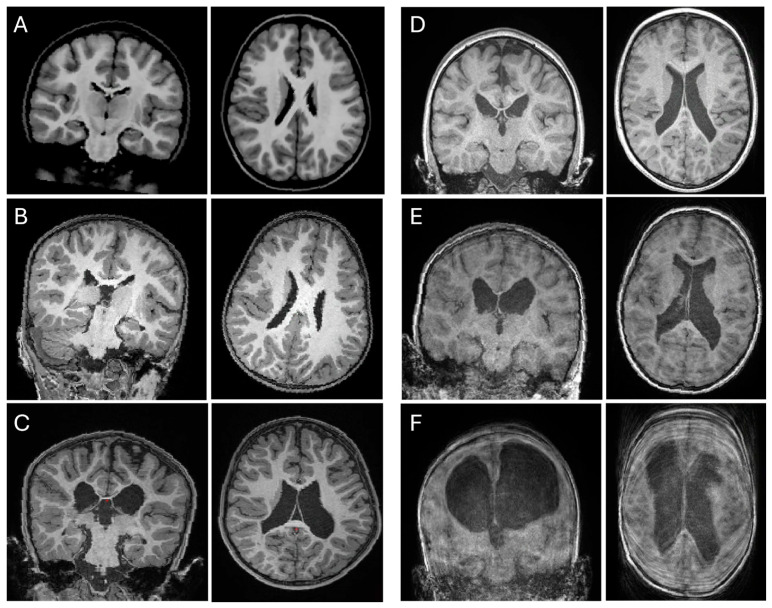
Representative coronal (left) and axial (right) T1-weighted images of *n* = 5 children that tolerated the non-sedate MRI, in order of degree of motion artifact (Child (B–F); child (F) with the highest degree of artifact). A neurotypical NIH template (4.5–8.5 y) is designated Child (A) for reference. Structural characterizations are described in Table 2.
